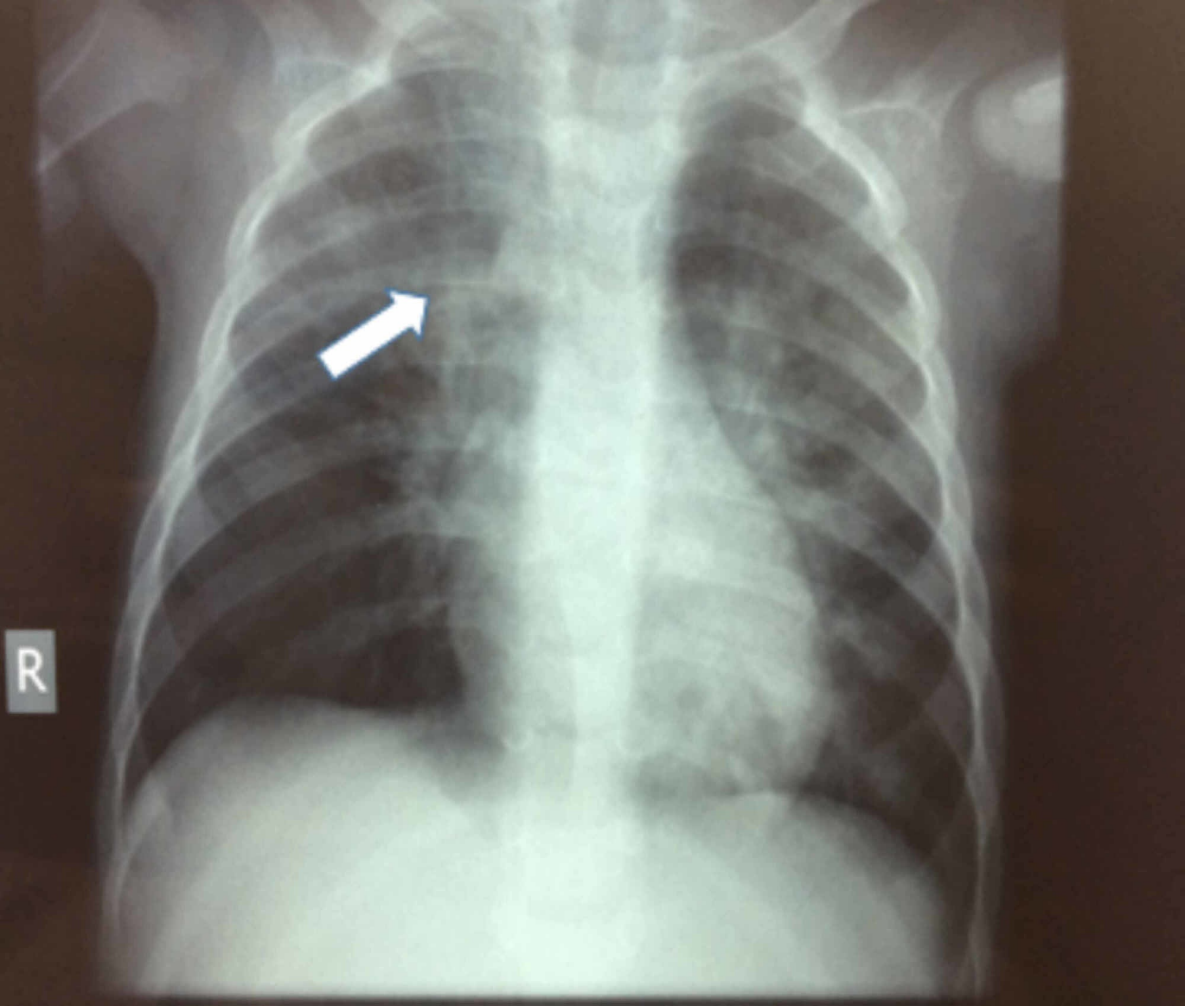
Patient's chest X-ray. Chest X-ray in admission showing mild infiltration
